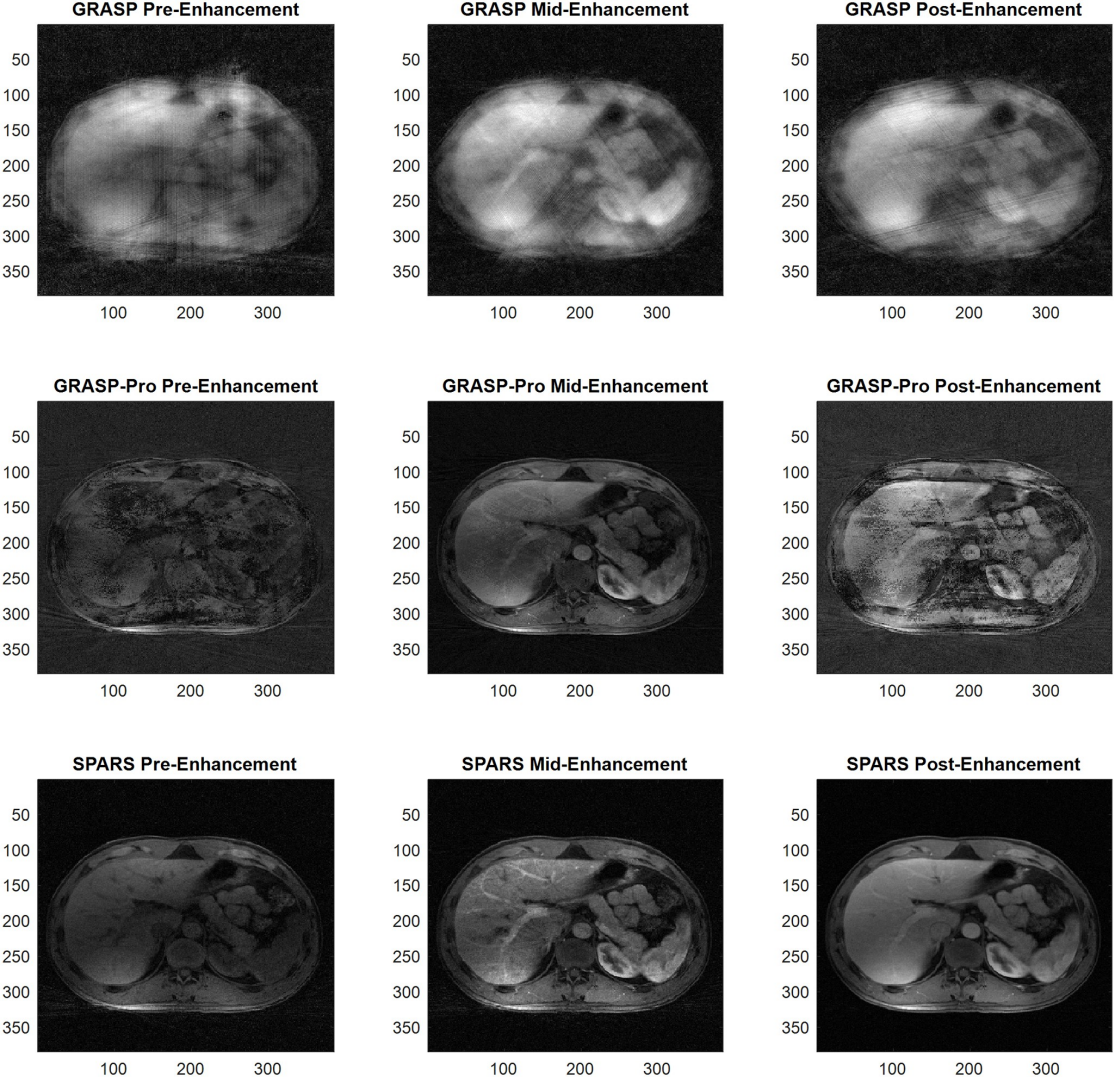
Reconstructed images of in-vivo human liver DCE-MRI for dataset 1. Comparison of GRASP, GRASP-Pro, and SPARS. Select timepoints are shown before, during, and after signal enhancement.
